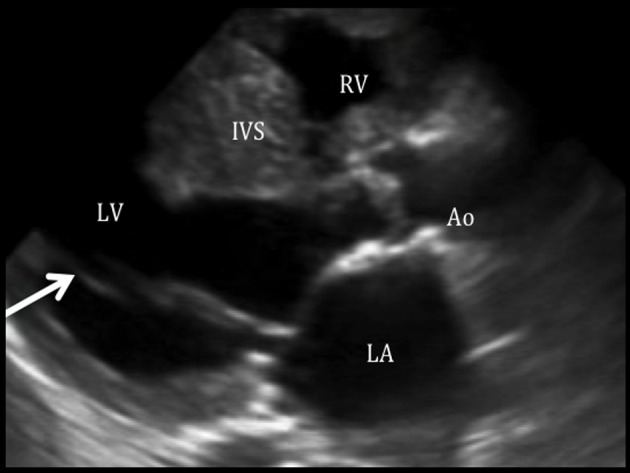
Parachute mitral valve with mitral stenosis on parasternal long axis view. Parasternal long axis view shows both chordae are attached to a single point (arrow), consistent with a parachute mitral valve, giving rise to a mitral stenosis. The left atrium (LA) is dilated with an LA volume index of 45 mL/m^2^. In this view, the aortic valve seems to be thickened. RV: right ventricle; IVS: interventricular septum; LV: left ventricle; Ao: aorta; LA: left atrium.
